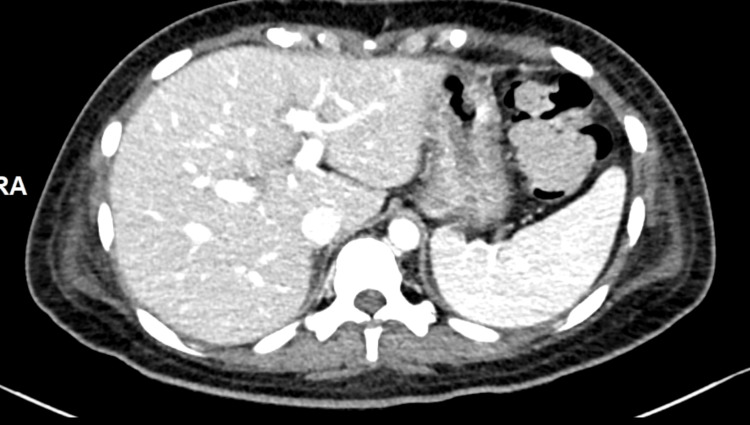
CT abdomen and pelvis with contrast (axial) Axial contrast-enhanced CT of the abdomen and pelvis demonstrating hepatomegaly with possible fatty infiltration and perihepatic ascites. CT: computed tomography
